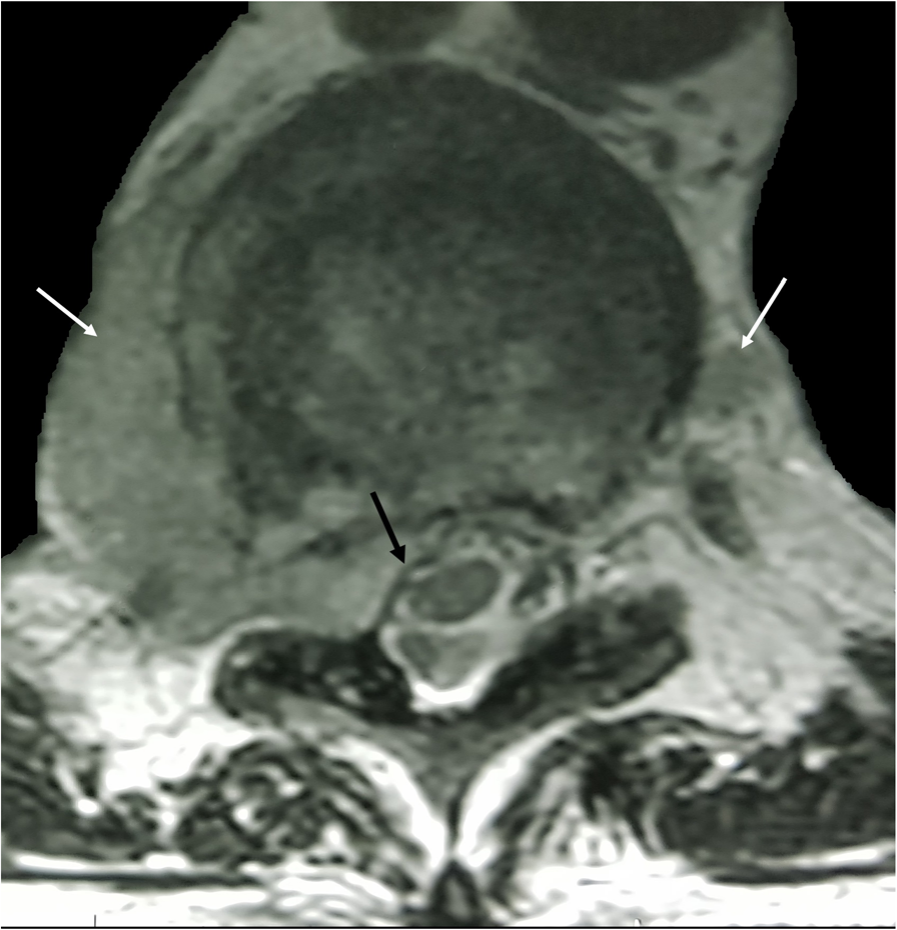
T2-weighted MRI demonstrating bilateral posterior mediastinal masses (white arrows) consistent with extramedullary haematopoiesis. The soft tissue has intruded the right neural exit foramen and into the vertebral canal, causing effacement and mild displacement of the thoracic cord (black arrow)
